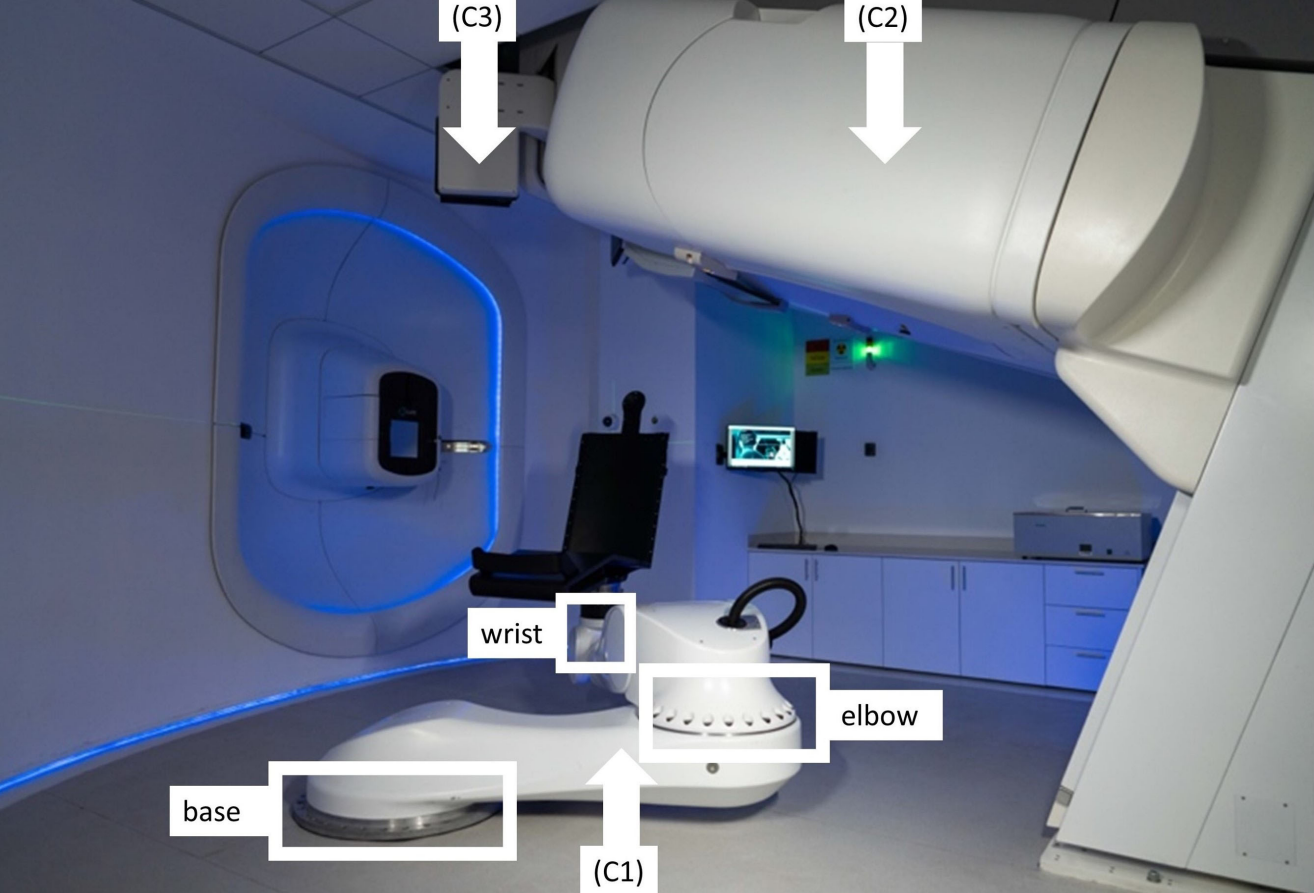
The P-ARTIS system consists of a patient positioning system (PPS) (C1) with mounted patient support (a chair); vertical 4DCT (C2) and X-ray orthogonal 2D system (C3).
